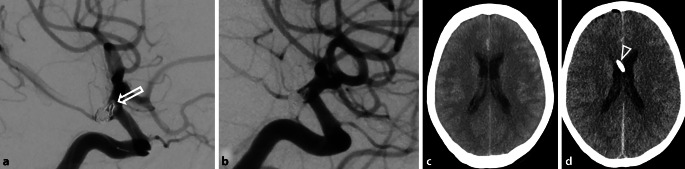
A patient with a ruptured posterior communicating artery (PCOM) aneurysm treated with coiling. a After coiling, an appositional thrombus is observed at a coil loop at the aneurysm base (arrow). b Resolution of the thrombus after tirofiban administration. c CT scan showing bilateral aSAH before coiling and EVD placement. d CT scan after EVD placement (*arrowhead*), coiling, and tirofiban administration, without signs of re-hemorrhage
